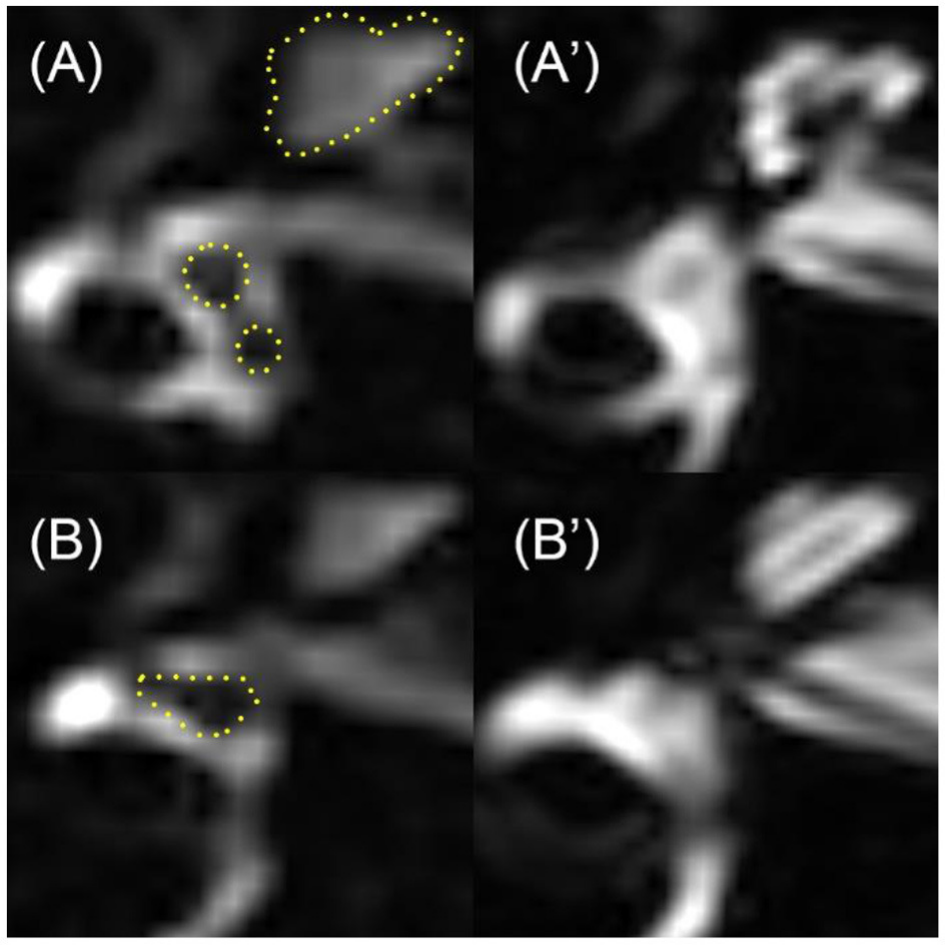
An example of the EH in an UISSNHL patient. This patient belonged to LF-G. 3D-FLAIR images on the left were compared with T2 images on the right. The evaluation was as follows: cochlea-grade I (three points). The cochlea in (A), especially the apical part of each turn, was smaller than the highlighted line, which was the outline of cochlea in T2 sequence in (A′). Vestibule-grade I (four points) and semicircular canals-grade I (five points): because the black area in (B) is over one-third of the whole ampulla, shown in (B′). Therefore, the sum EH level of this patient was 12 points.
